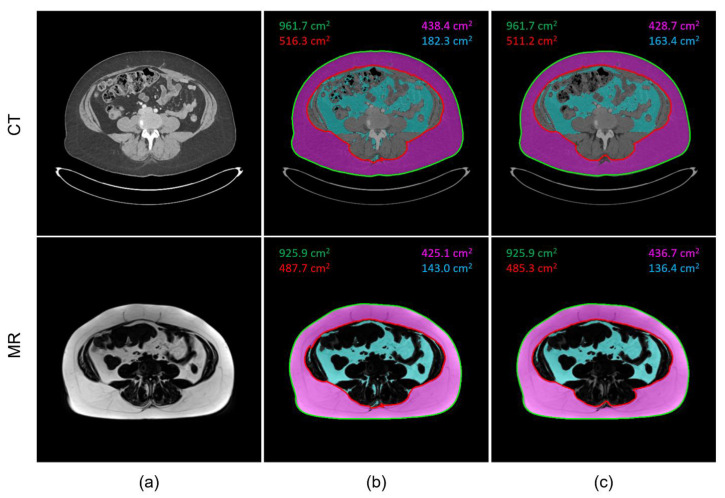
Qualitative comparison of CT (top row) and MR (bottom row) image segmentation and fat quantification based on the proposed approach. (a) An example matched image pair at the level of L4–L5 vertebrae. (b) Computer automatically generated abdominal wall boundary and adipose tissue masks, where the outer contour is displayed in green and the inner contour in red; SAT and VAT regions are shown in magenta and cyan color masks, respectively. (c) The outer and inner contours as well as SAT and VAT masks after the manual corrections.
